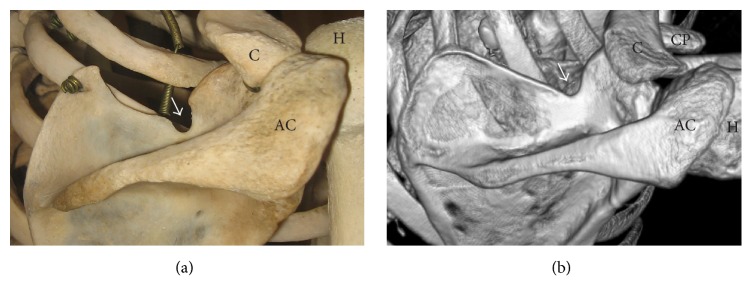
Posterior surface of the scapula: arrow: suprascapular notch, AC: acromion, C: clavicle, CP: coracoid process, and H: humerus. (a) The dry scapula; (b) three-dimensional volume rendering (VR) multidetector computed tomography (MDCT).
